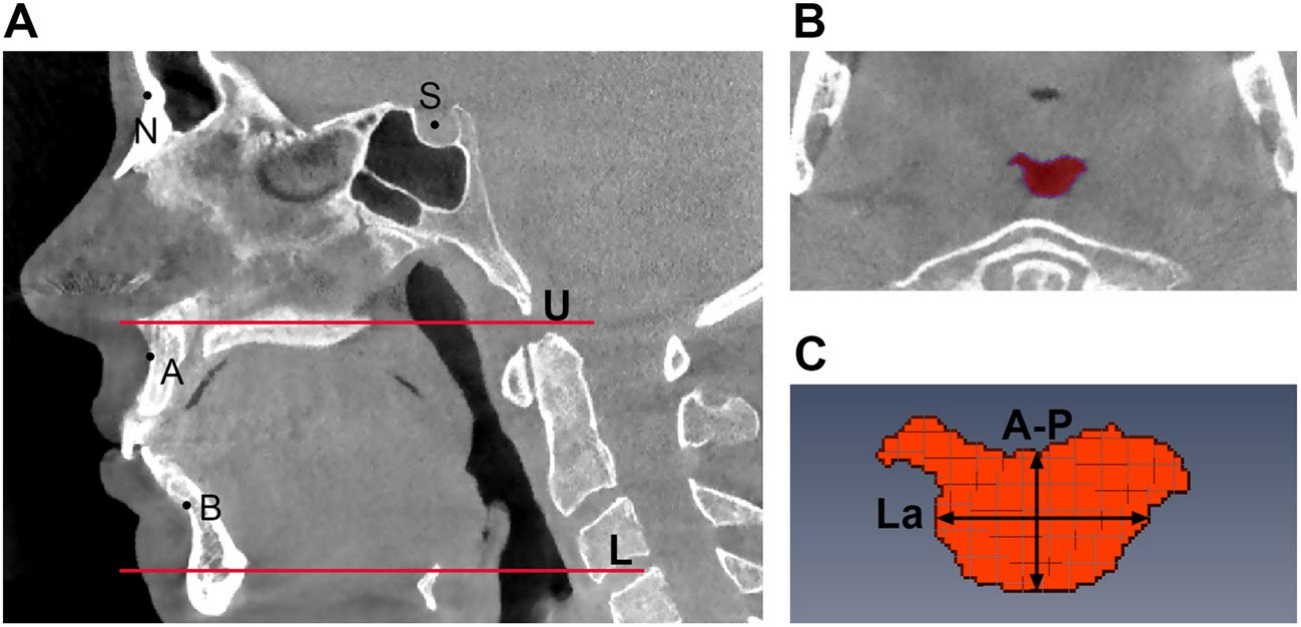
Craniofacial landmarks and measurements of the upper airway dimensions based on cone beam computed tomography (CBCT) imaging. A N = nasion, S = sella, A = subspinale, B = supramentale, U= the upper boundary of the upper airway (hard palate plane), L = the lower boundary of the upper airway (plane cross the base of the epiglottis) on the mid-sagittal plane. B The minimal cross-sectional area of the upper airway (CSAmin) on the axial plane. C The measurement of the anteroposterior dimension (A-P) and the lateral dimension (La) of the CSAmin
